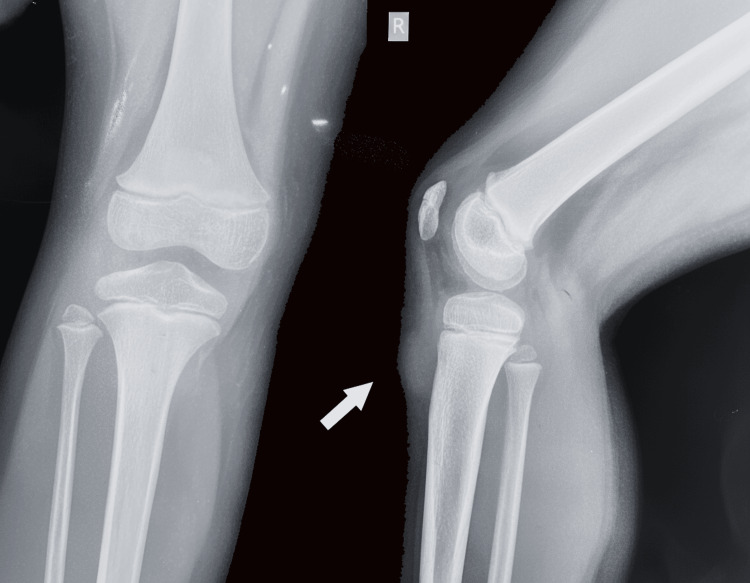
X-ray of the knee demonstrating a clear margin soft tissue enlargement, with intact bone and no periosteal reaction (arrow).
